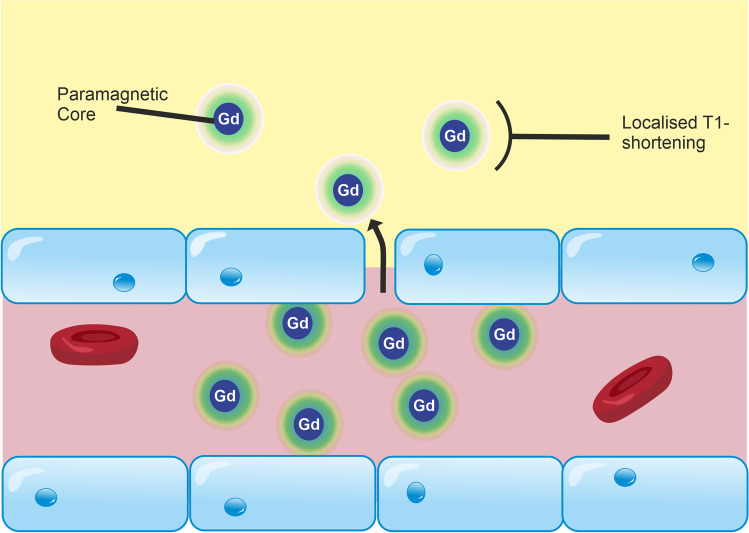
Leakage of GBCA across the BBB in DCE-MRI. DCE-MRI uses an intravenous injection of *T*_1_-shortening GBCAs. Under normal conditions, GBCA molecules are restricted from the CNS by the BBB. Inflammation reduces the junctional expression of TJ proteins in endothelial cells, which increases the size of molecules that are able to cross the BBB. As a result, GBCA molecules are able to permeate the CNS. These molecules consist of a paramagnetic core, which interacts with adjacent water molecules to increase the rate of *T*_1_ relaxation, which is detected as a signal enhancement on *T*_1_-weighted MRI. By scanning dynamically and applying kinetic models to the change in signal over time, the leakage rate of GBCA can be estimated and used as a measure of paracellular integrity
